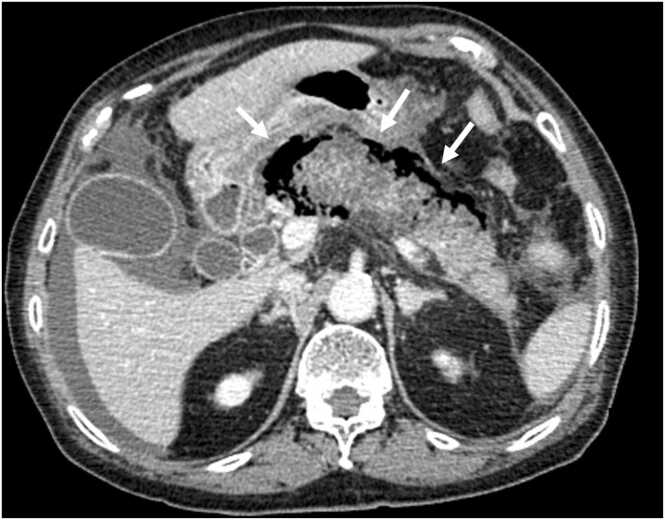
Contrast-enhanced computed tomography scan of an 83-year-old woman showing an enlarged pancreas and peripancreatic gas.
